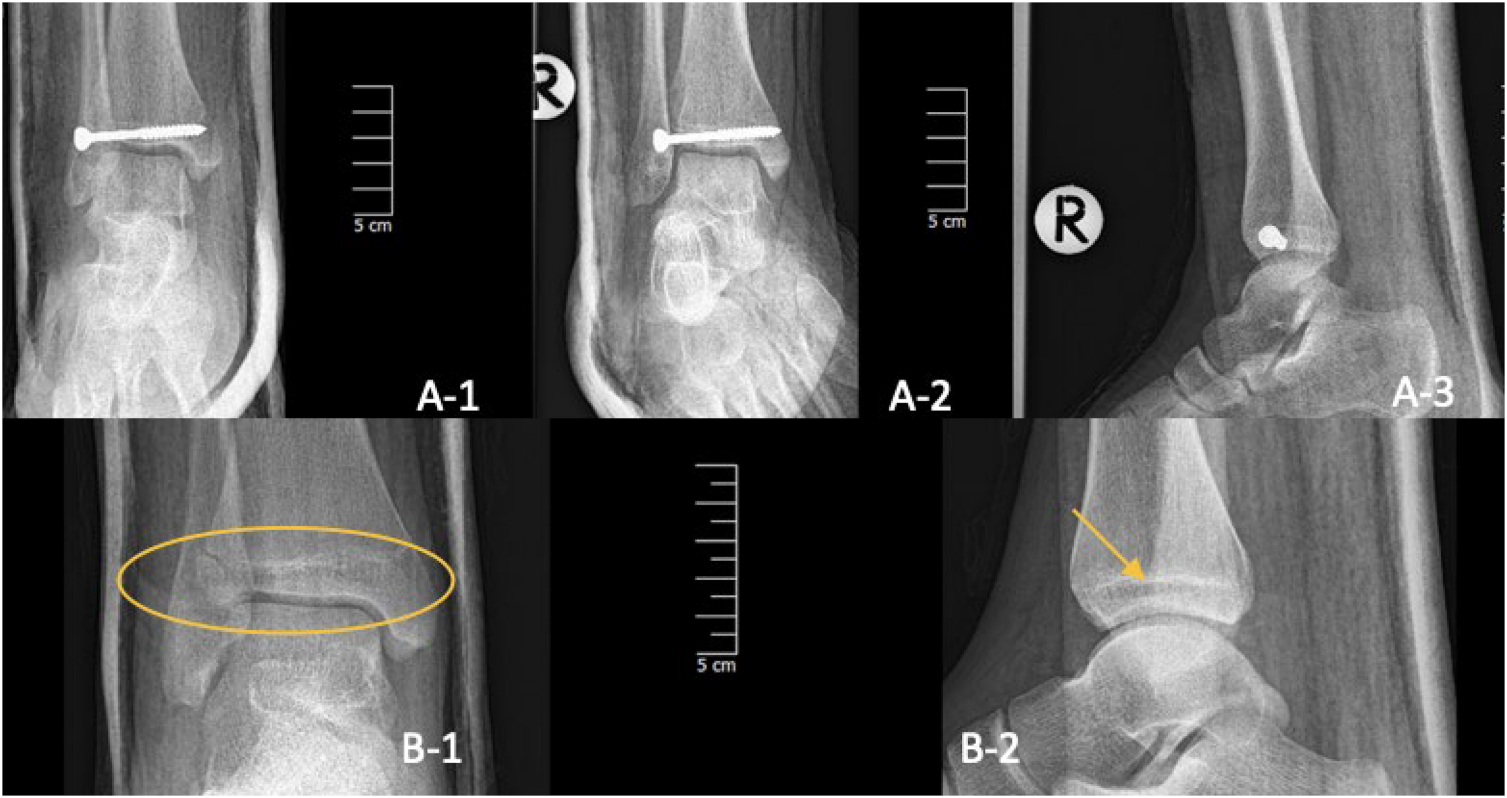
Three-plane radiographs of SH-III fracture (Tillaux) one-day post-op in one child treated with metal (A-1/2/3) and two-plane radiographs in another treated with PLGA implants (B-1/2). The PLGA inserts are marked with a yellow oval and arrow.
